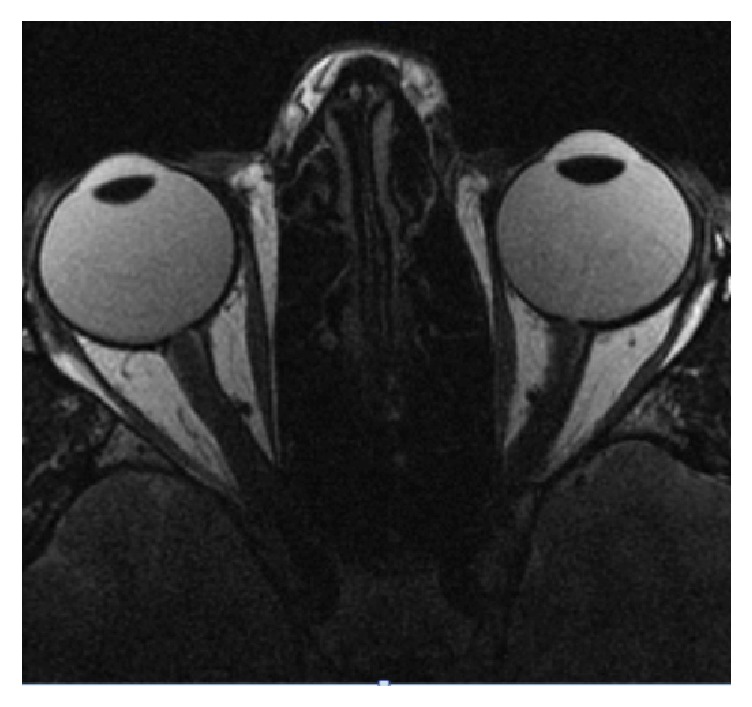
MRI scan of the orbits depicting normal extraocular muscles bilaterally without evidence of fibrosis or atrophy.
